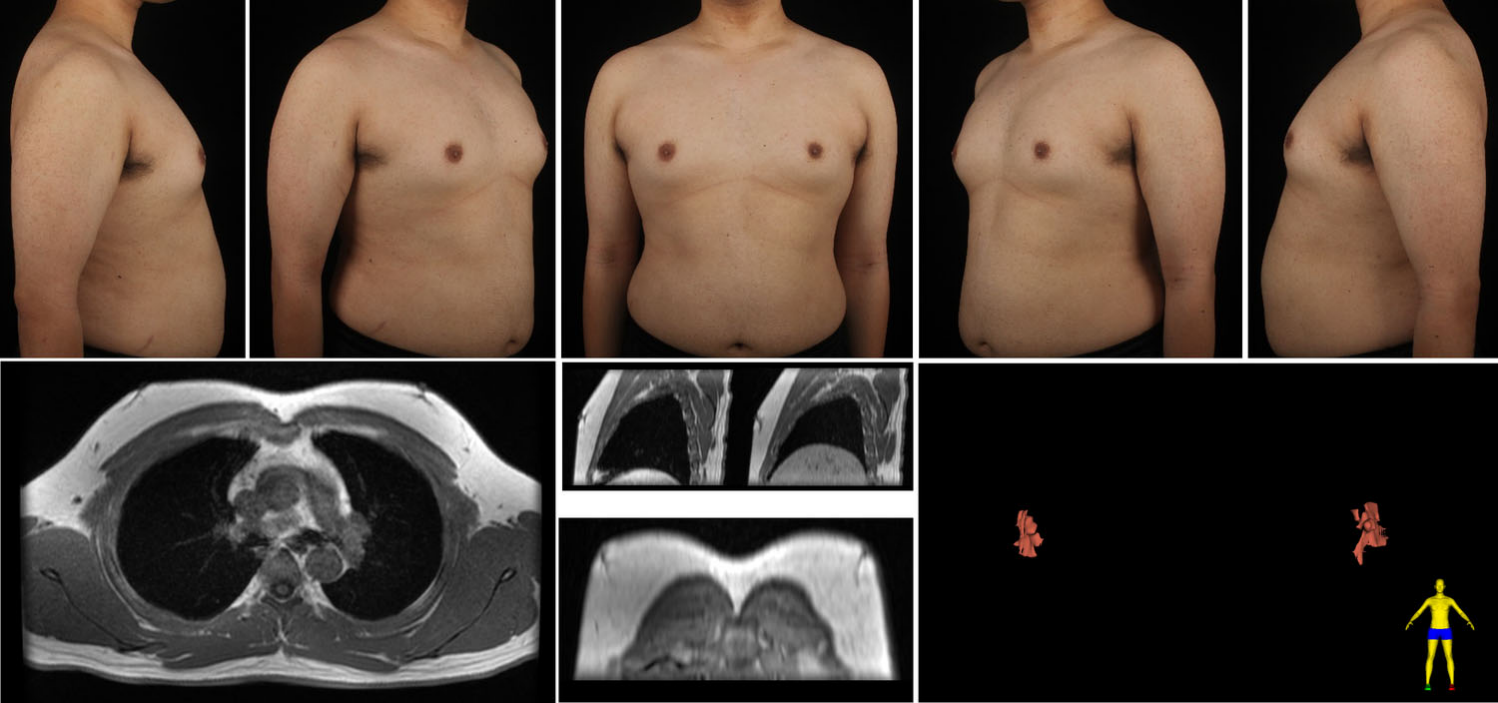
Type 1A gynecomastia. Preoperative chest appearance (upper) and breast MR images (lower) of a 29-year-old man with type 1A gynecomastia
